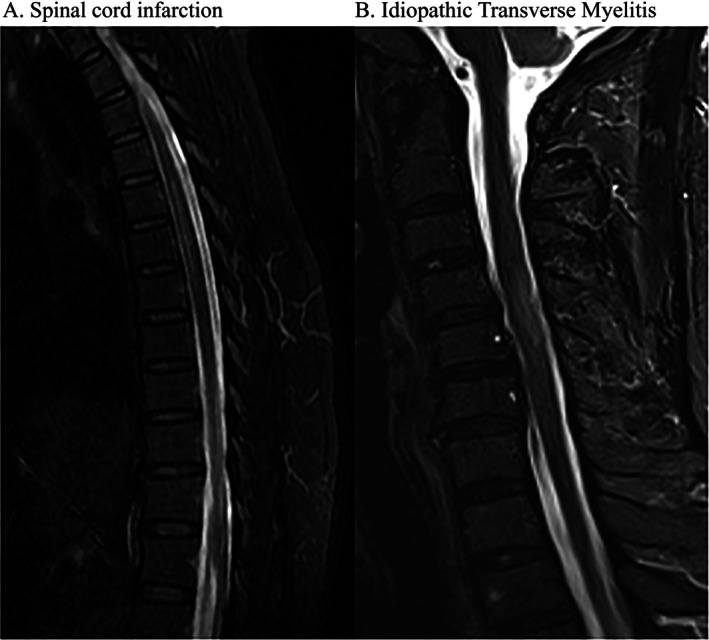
The linear lesion in SCI versus the patchy lesion in ITM on sagittal spinal MRI. (A) The linear lesion (red arrow) depicted on the sagittal spinal MRI of a patient (Case 11) diagnosed with definite spinal cord infarction. (B) The patchy lesion (red arrow) depicted on the sagittal spinal MRI of a patient (Case 9) diagnosed with ITM. ITM, idiopathic transverse myelitis; MRI, magnetic resonance imaging; SCI, spinal cord infarction.
